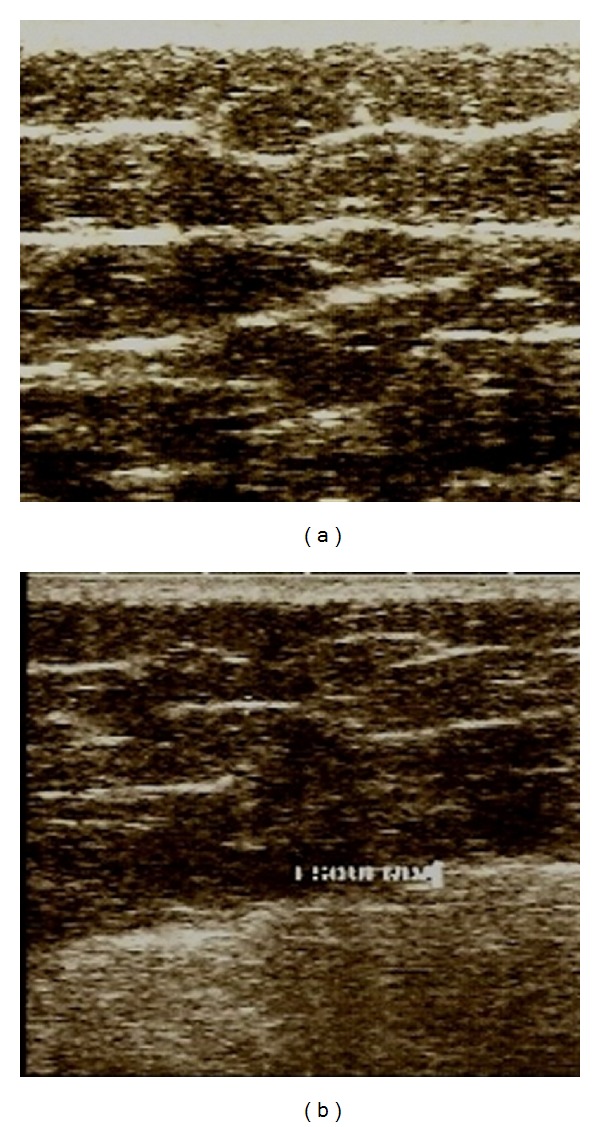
Ultrasonography before and after 10 sessions.
